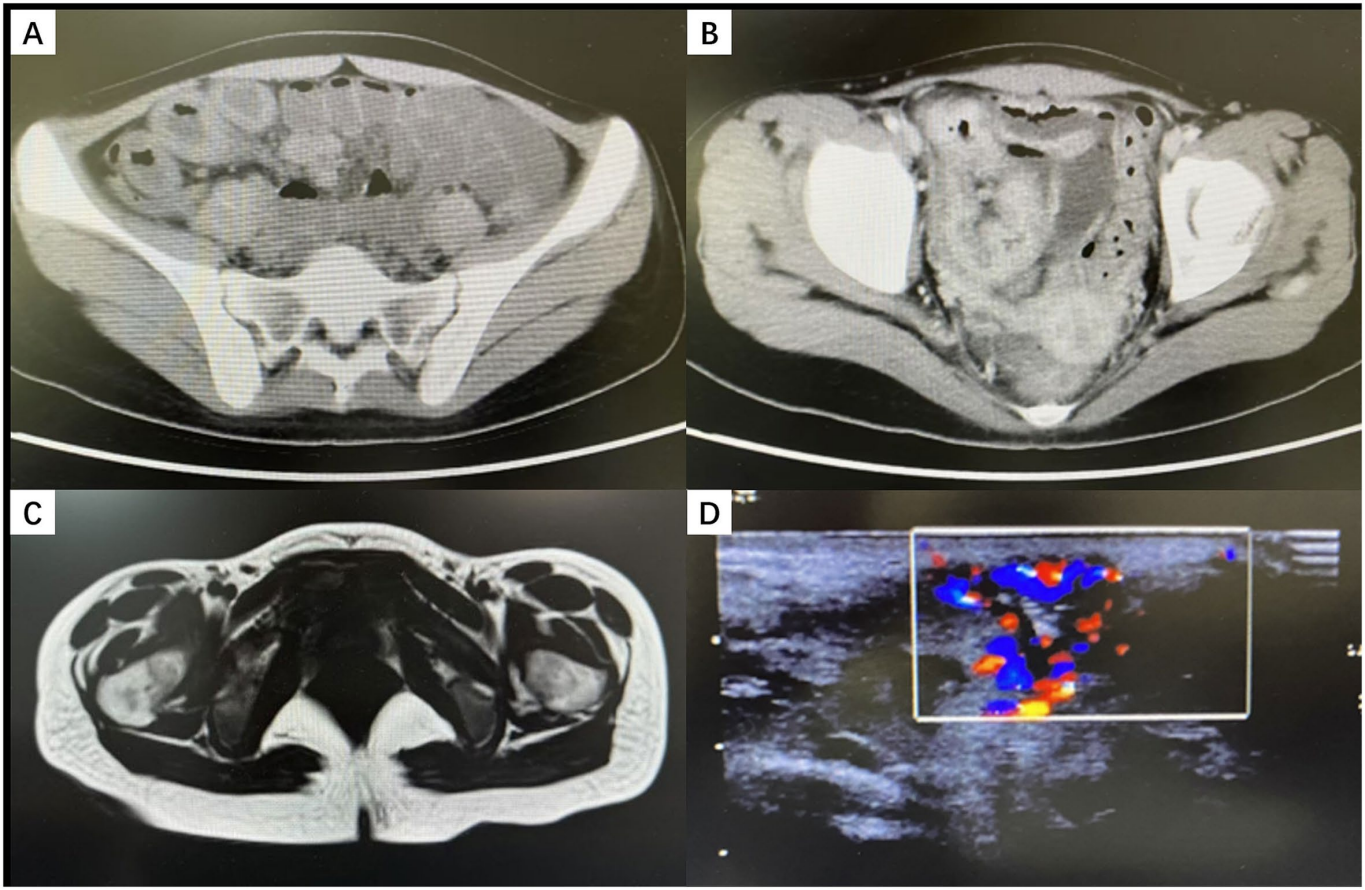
Imaging findings (A,B) Contrast-enhanced abdominal CT showing marked thickening and enhancement of the terminal ileum. (C) Contrast-enhanced pelvic MRI showing post–incision and drainage changes of a left vulvar abscess, with an abnormal signal posterior to the left vaginal introitus, suggesting a residual abscess. (D) Perineal ultrasonography revealing a left-sided perineal abscess with abundant blood flow.
